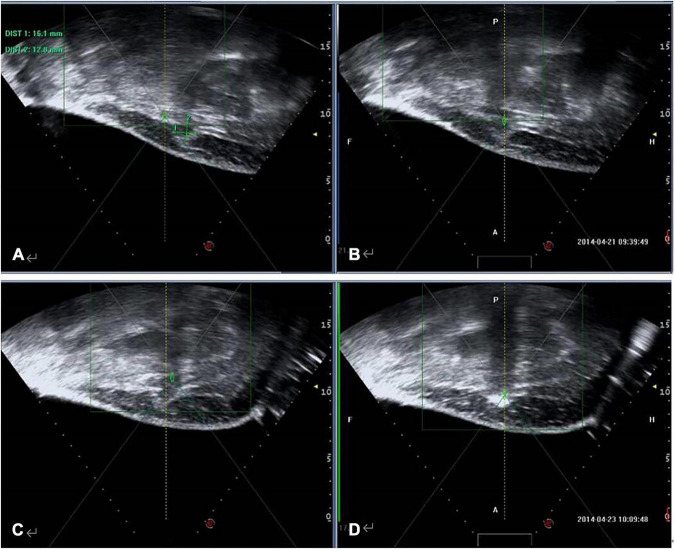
Ultrasound imaging in a 35-year-old woman with AWE. Intraoperative ultrasound was used to measure and locate the AWE lesion. (A) Before HIFU ablation, sagittal-guided ultrasound showed a low echogenic nodule of approximately 16.1 × 12.8 mm in the right subcutaneous muscle layer of the abdominal wall incision (white arrow). (B) Focused ultrasound focus localization was displayed before ablation (white arrow). (C) During the operation, ultrasound energy of fixed-point emission (white arrow). (D) The focus position showed a massive hyperechoic change (white arrow), but there was no significant echo change in the superficial tissue of the lesion.
